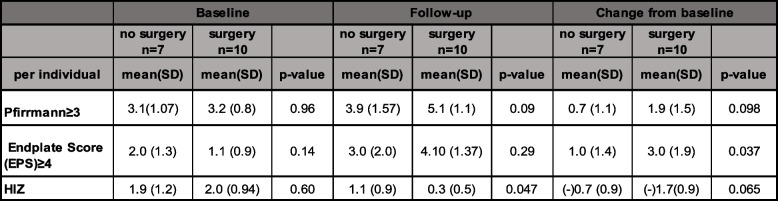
Magnetic resonance imaging parameters at baseline and follow-up for patients with and without fusion surgery during the follow-up period
